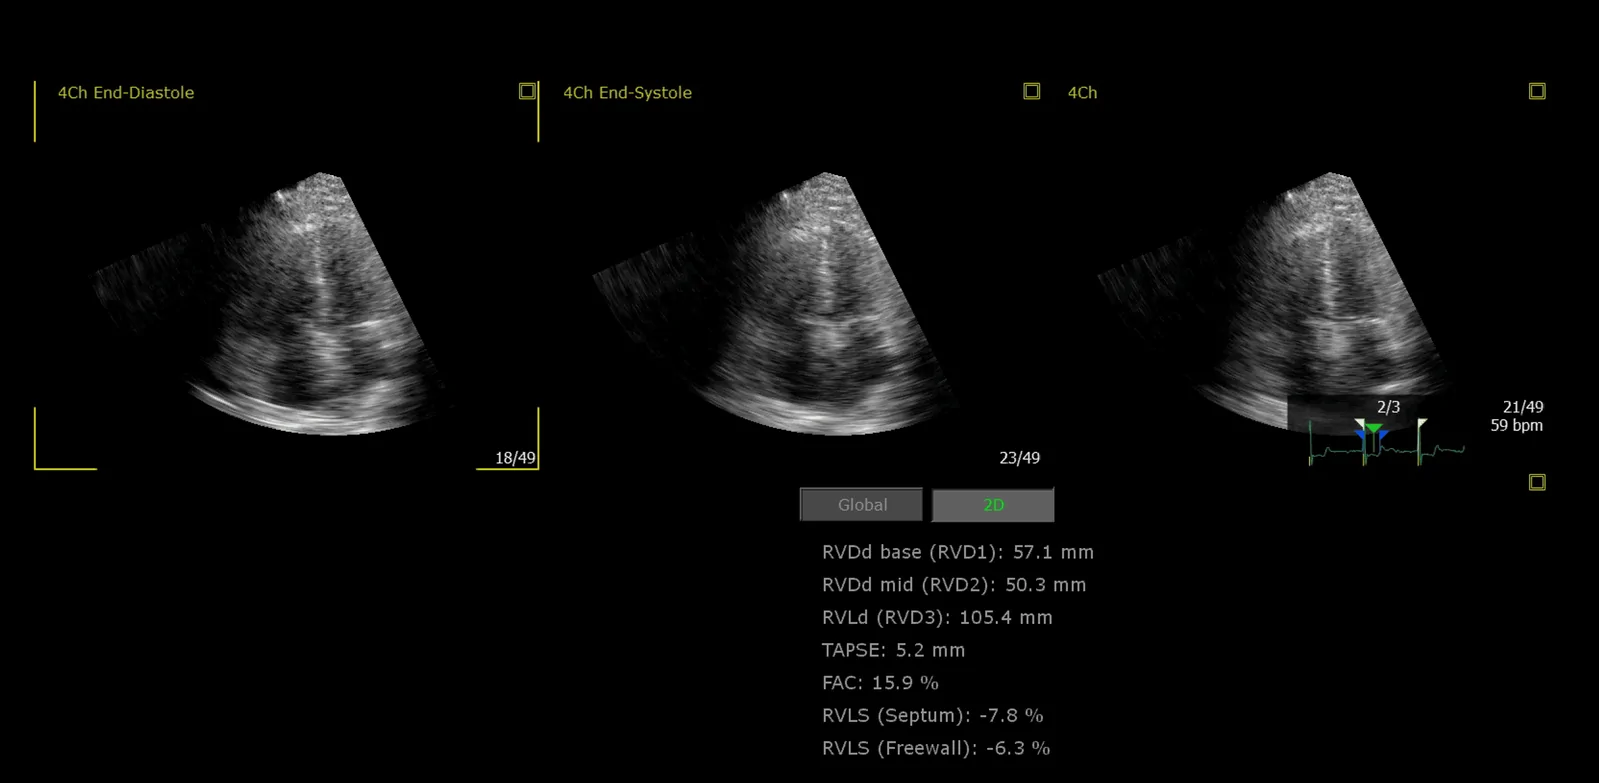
Quantitative echocardiographic assessment of right ventricular size and systolic function Quantitative two-dimensional echocardiographic assessment demonstrating severe right ventricular enlargement, with a basal diameter (RVD1) of 57.1 mm, mid-cavity diameter (RVD2) of 50.3 mm, and longitudinal diameter (RVD3) of 105.4 mm. Right ventricular systolic function was severely impaired, with a tricuspid annular plane systolic excursion (TAPSE) of 5.2 mm and fractional area change (FAC) of 15.9%.
